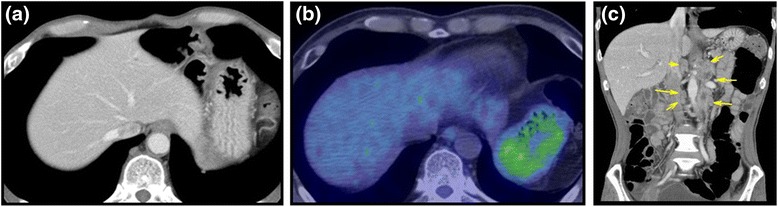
Enhanced CT and PET-CT. CT (a) and PET-CT (b) failed to detect the tumor in the liver. CT revealed multiple swollen abdominal lymph nodes (*arrows*) (c)
